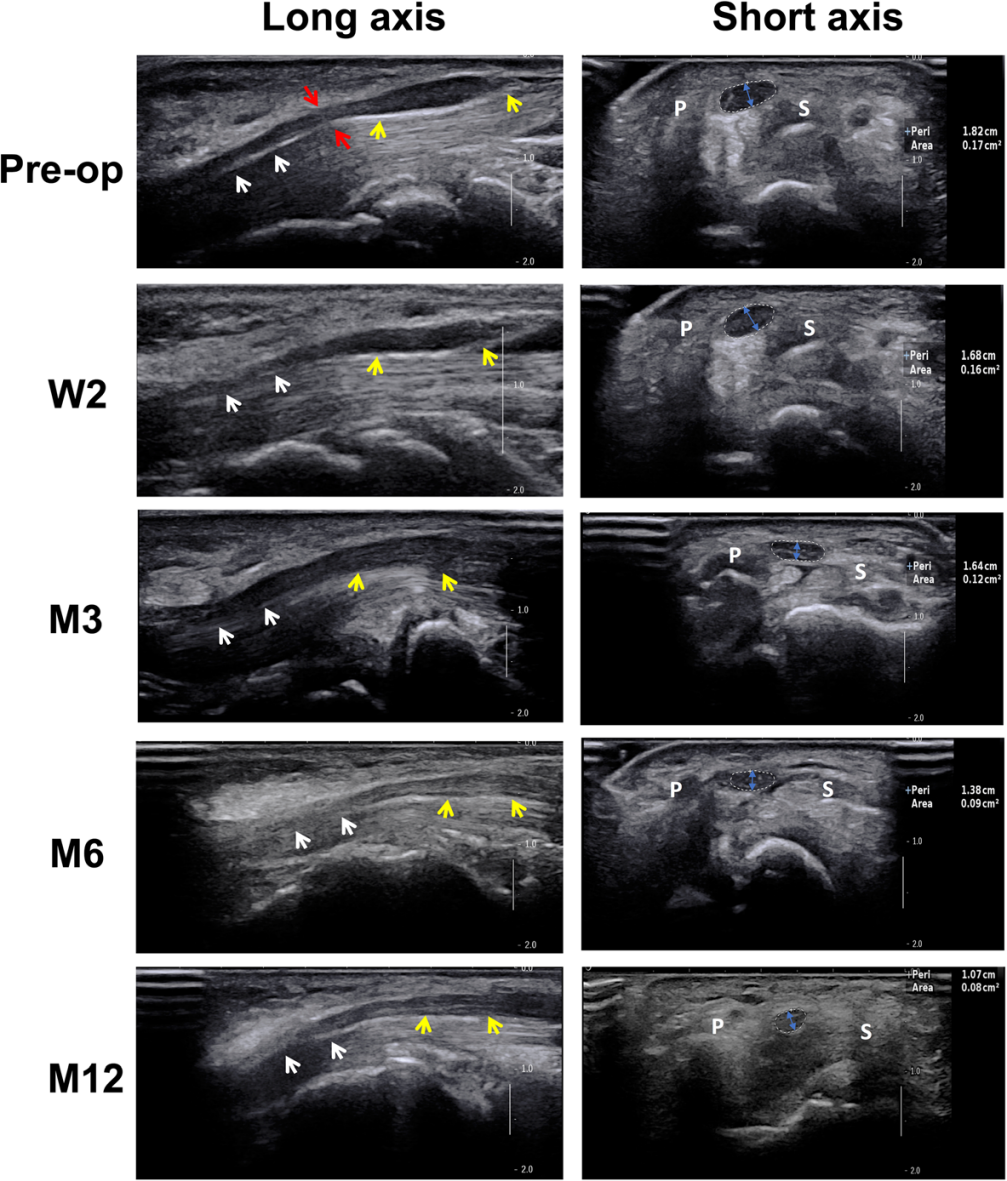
High frequency ultrasonic imaging of carpal tunnel from right hand of a 48-year-old man with severe CTS. The long axis figures of median nerve were showed in left column. The white arrow indicated the median nerve at the distal end of the carpal tunnel, and the yellow arrow indicated the median nerve at the proximal end of the carpal tunnel. Between the both yellow arrow was the edema length (EL) of median nerve. The red arrow pointed at the compression site. Representative figures on the short axis plane at the inlet of the carpal tunnel (the level of distal wrist crease) were showed in the right column. The cross-sectional area (CSA) of the median nerve was delineated by dashed line and the values were showed on the right side. The anteroposterior diameter (D) of median nerve was showed by blue double arrow line. P and S indicated pisiform and scaphoid separately. Pre-op: 3 days pre-surgery; W2: 2 weeks post-surgery; M3: 3 months post-surgery; M6: 6 months post-surgery; M12: 12 months post-surgery
